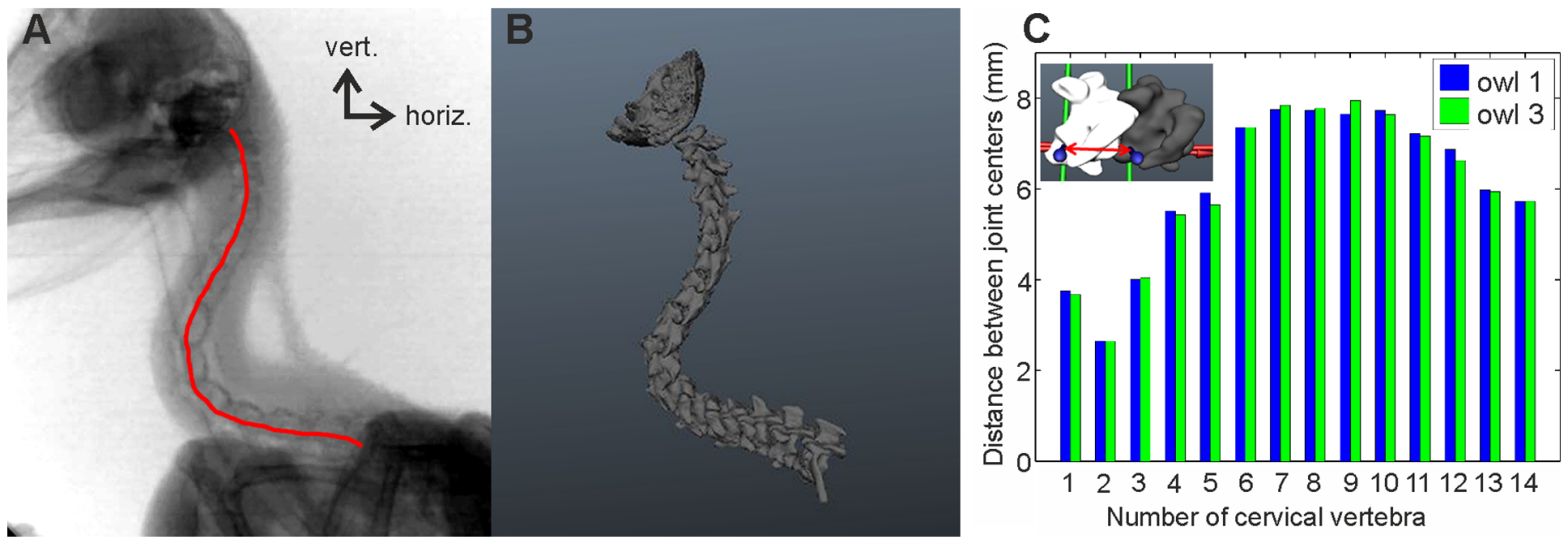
The cervical column in natural posture. A) X-ray image of owl 1 in the setup shown in Figure 2 displaying the natural posture. Note the S-shaped neck. The red line approximates the curvature of the cervical spine and was used for the realignment. B) Shape of the cervical column when the owl assumes its natural posture after realignment of the cervical vertebrae. The shape was extracted from the image shown in A). Note that the caudal part is almost horizontal, whereas the middle region is almost vertical. C) The distance between joint centers. The inset shows how the distance was measured. Joint 0 refers to the join between occiput and atlas, joint 1 between atlas and axis and so on. Note that the highest values occur in the middle of the cervical column.
